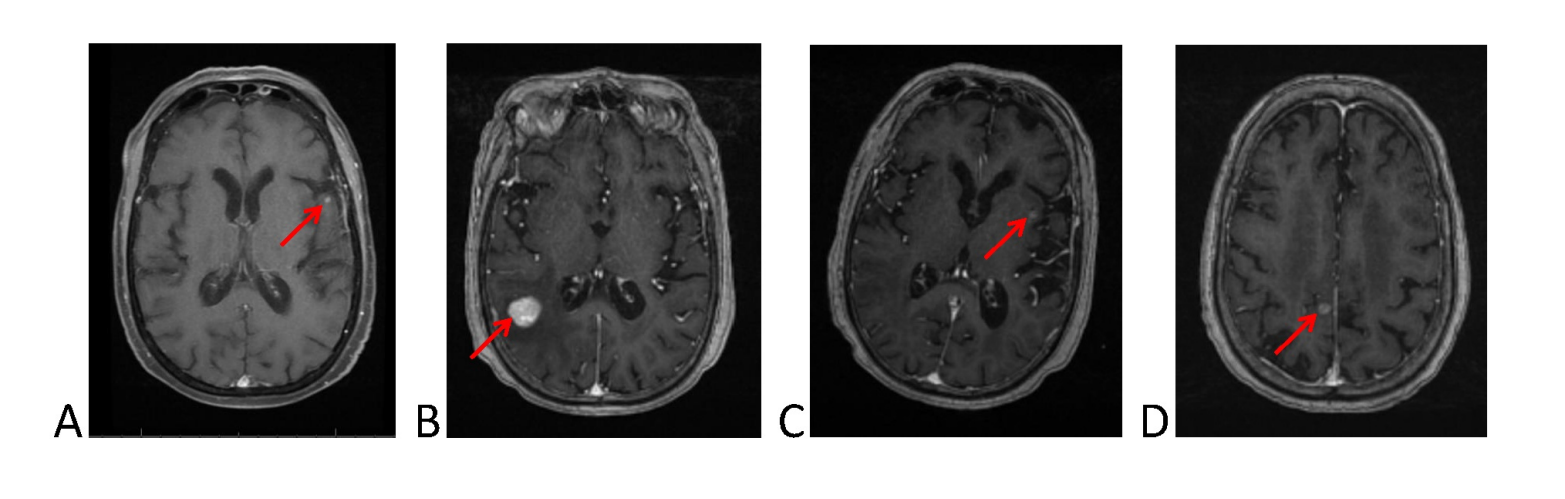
MRI scans of the brain of the patient at nine, 30, 34, and 44 months (images A, B, C, D, respectively) New brain metastases are highlighted by arrows. The patient had stereotactic radiosurgery for each new brain metastasis.
